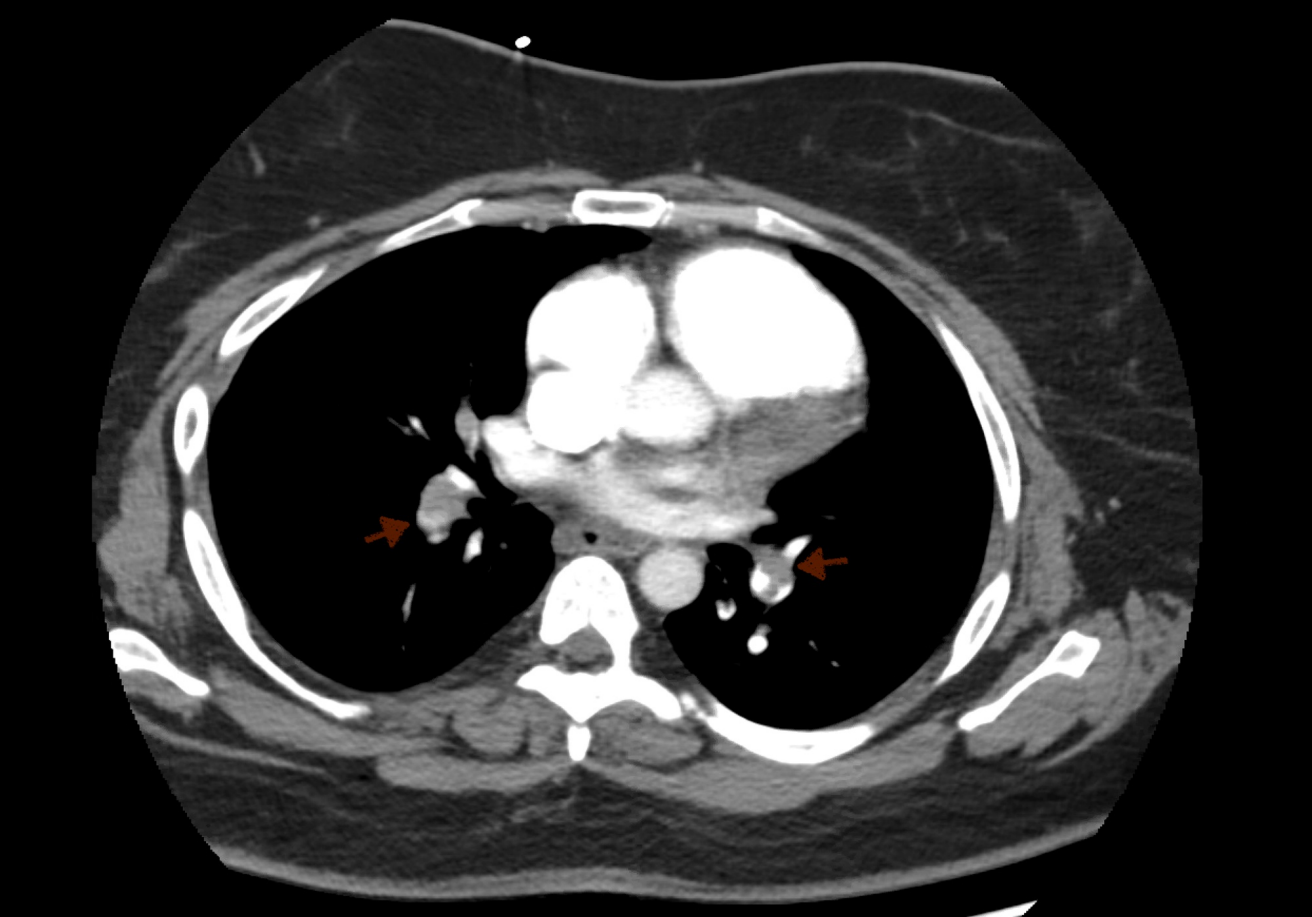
CT with pulmonary embolism protocol The image shows severe bilateral pulmonary embolism with associated right heart strain with compression of the left ventricle. Red arrows: pulmonary embolism CT: computed tomography
